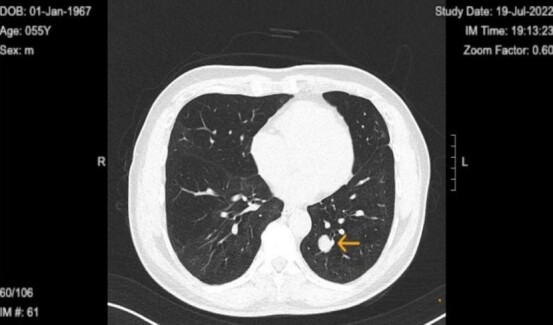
Chest computed tomography in 2022 revealed well circumscribed solitary lung tumor of 1.5 × 1.3 cm in size.
